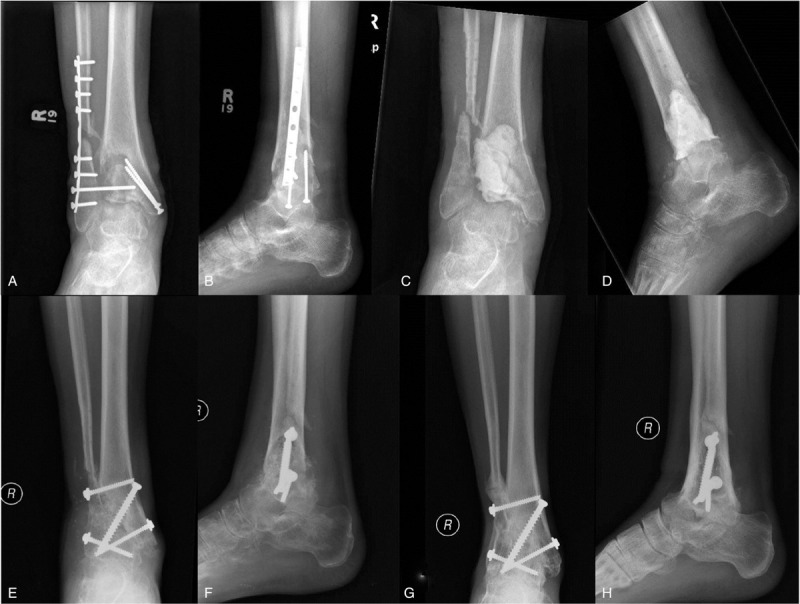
Infected ankle nonunion and Masquelet technique. Radiographs of 25-year-old male 6 months after open reduction and internal fixation of a work-related grade II open bimalleolar ankle fracture demonstrating an infected nonunion (A and B). He had ongoing medial wound drainage despite a deep wound washout and prolonged antibiotic therapy. Postoperative radiographs 6 weeks after complete hardware removal, irrigation and debridement, and insertion of an antibiotic cement spacer as the first stage of the induced membrane (Masquelet) technique (C and D). He received 6 weeks of intravenous culture-specific antibiotics and his draining wound healed within 3 weeks of this procedure. Initial postoperative radiographs following removal of the cement spacer and ankle fusion with Iliac Crest bone graft and platelet-derived growth factor (PDGF, Augment, Wright Medical) insertion (E and F). Follow-up radiographs 1 year after the second stage procedure demonstrated solid union of the ankle fusion (G and H). The patient was able to return to work as a landscaper.
